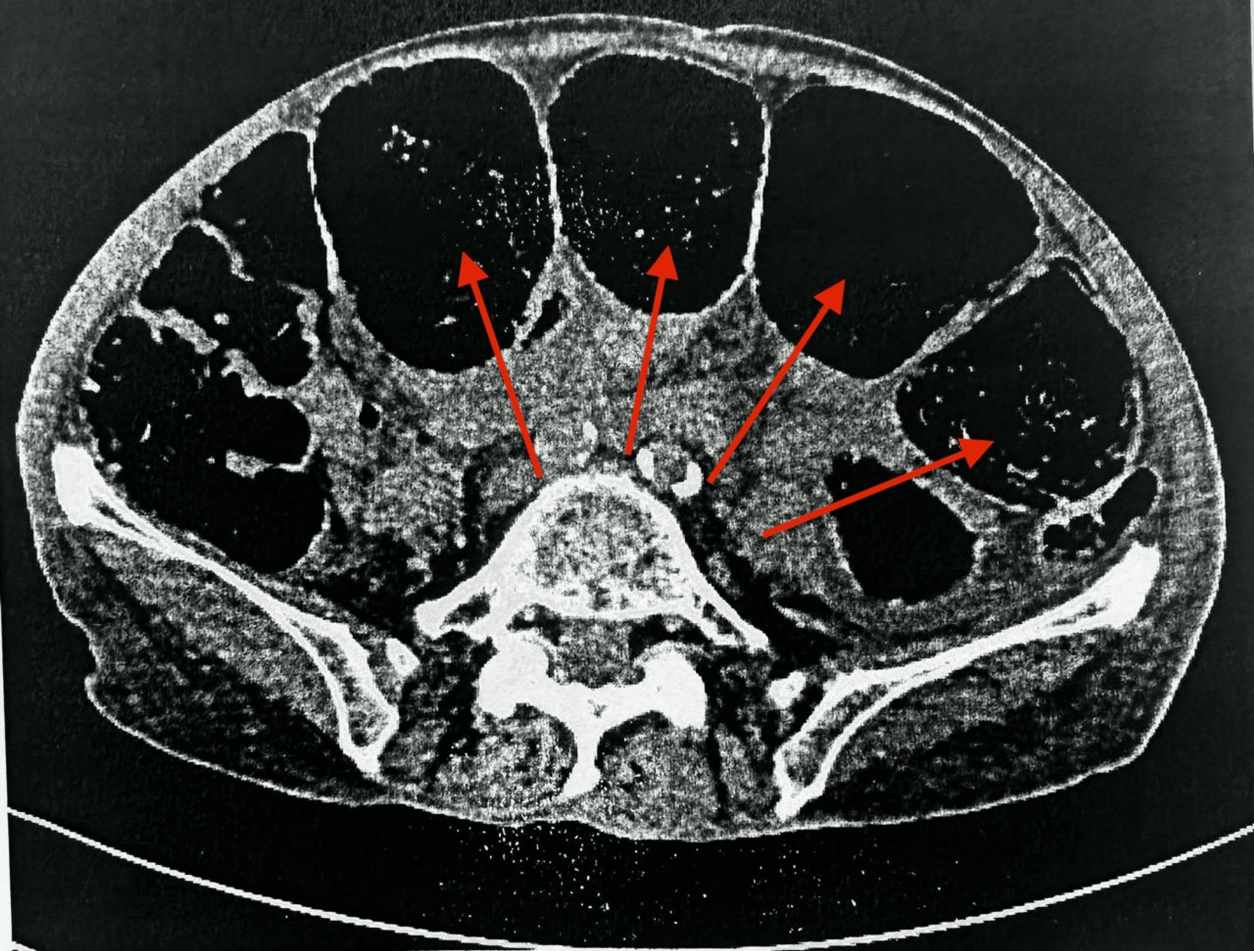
CT scan showing the marked gaseous distension of large bowel loops up to ~10 cm, consistent with large bowel obstruction. Arrows indicate distended bowel loops. CT: computed tomography
